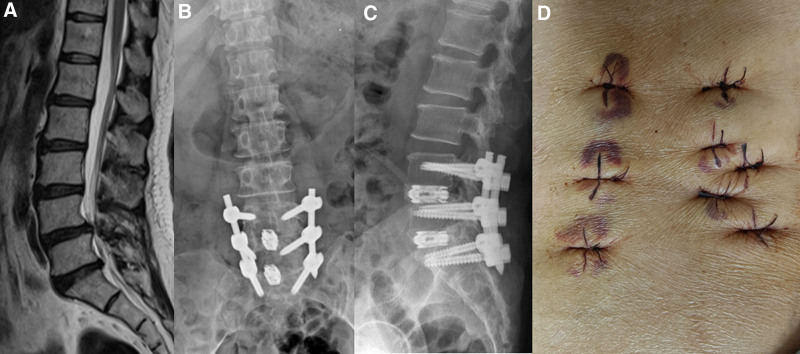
(A) Preoperative MR; (B) and (C) postoperative X-ray; (D) postoperative incision. MR = magnetic resonance.
